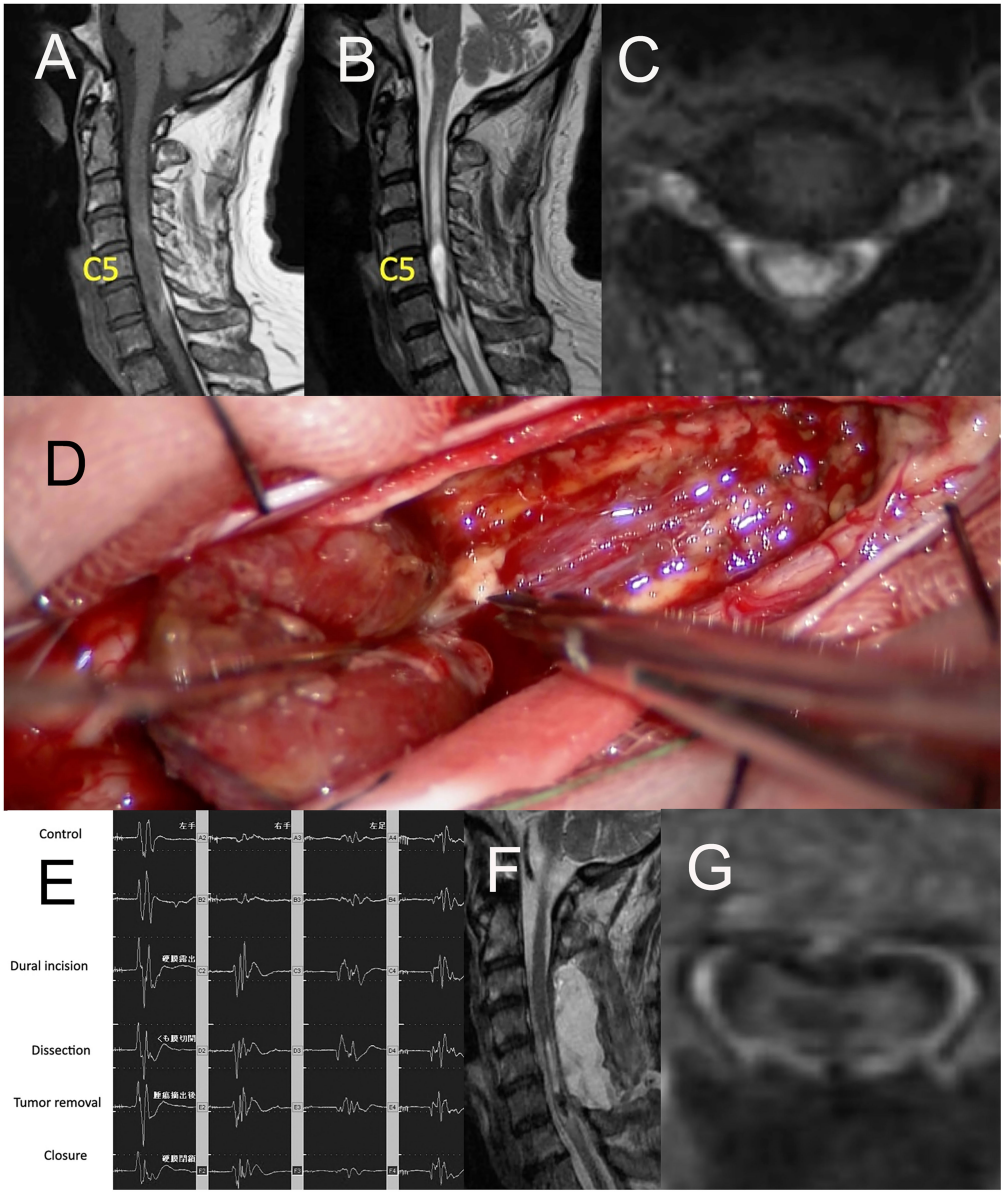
(A) Sagittal image of preoperative Gd-enhanced T1WI of MRI, (B) the axial image of preoperative T2WI of MRI, (C) the axial image of preoperative Gd-enhanced T1WI of MRI, (D) intraoperative photograph of tumor resection through midline myelotomy by posterior approach, (E) Ratio motor-evoked potential (MEP) of both UE and LE ranged from 91 to 108%, (F) Sagittal image of postoperative Gd-enhanced T1WI of MRI, (G) the axial image of postoperative Gd-enhanced T1WI of MRI. Gd, gadolinium; TIWI, T1-weighted image; MRI, magnetic resonance imaging; T2WI, T2-weighted image; UE, upper extremity; LE, lower extremity.
